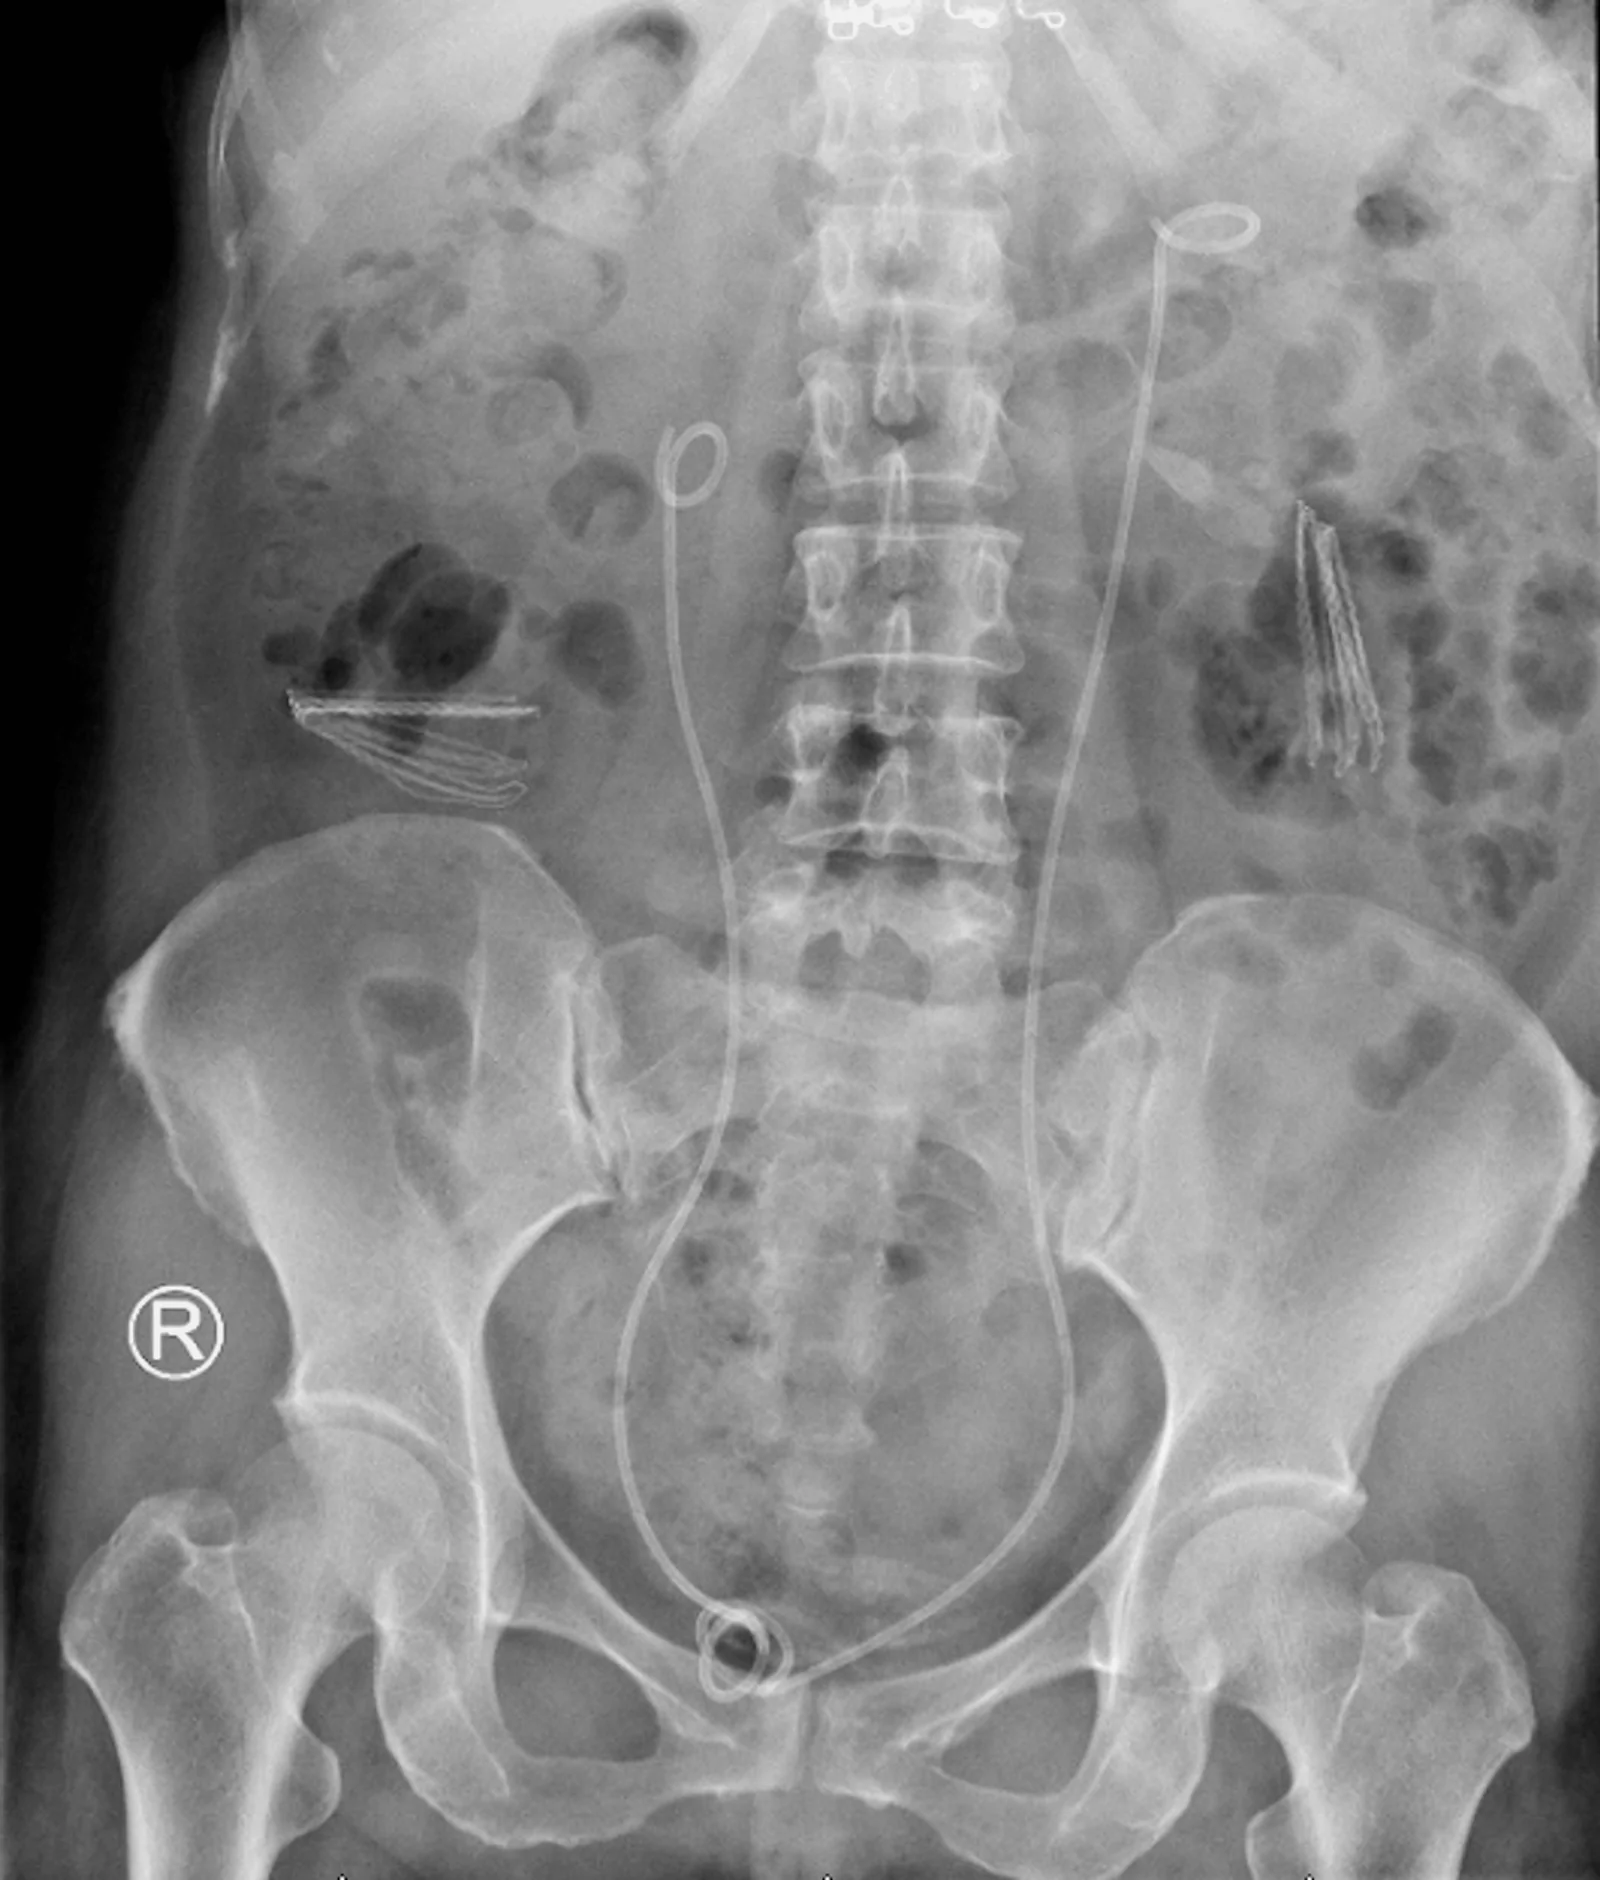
Postoperative KUB radiograph showing no visible residual stones in either ureter. KUB: Kidney-ureter-bladder.
